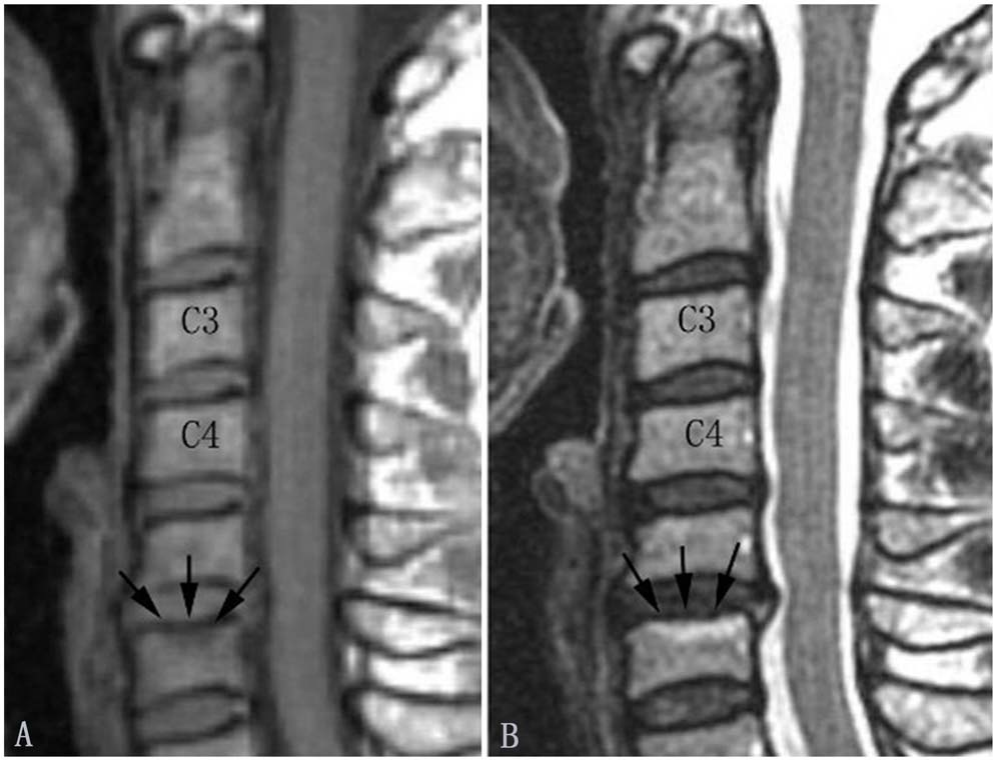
Modic type I changes in the cervical spine (indicated by arrows). Modic type I changes appear hypointense on T1-weighted images (A) and hyperintense on T2-weighted images (B).
